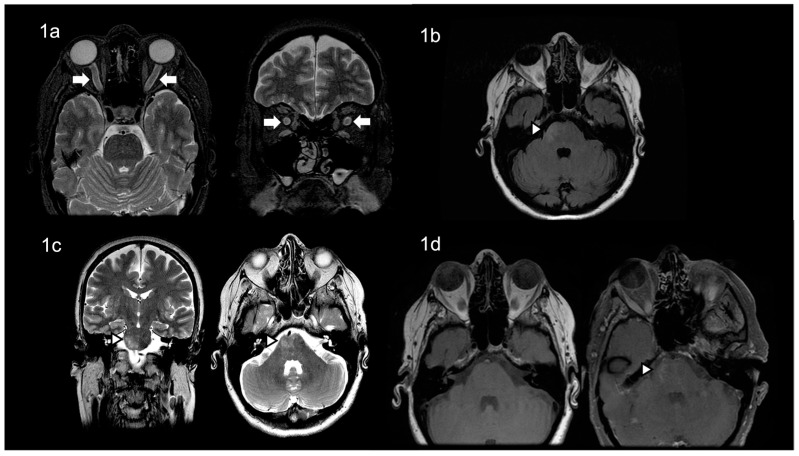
The patient’s brain MRI revealed, in T2 images, (a) mild thickening of the bilateral optic nerves, and (b,c) high-intensity lesions in the medial and right lateral pons, in T2/FLAIR images, (d) with contrast enhancement of the above lesions.
